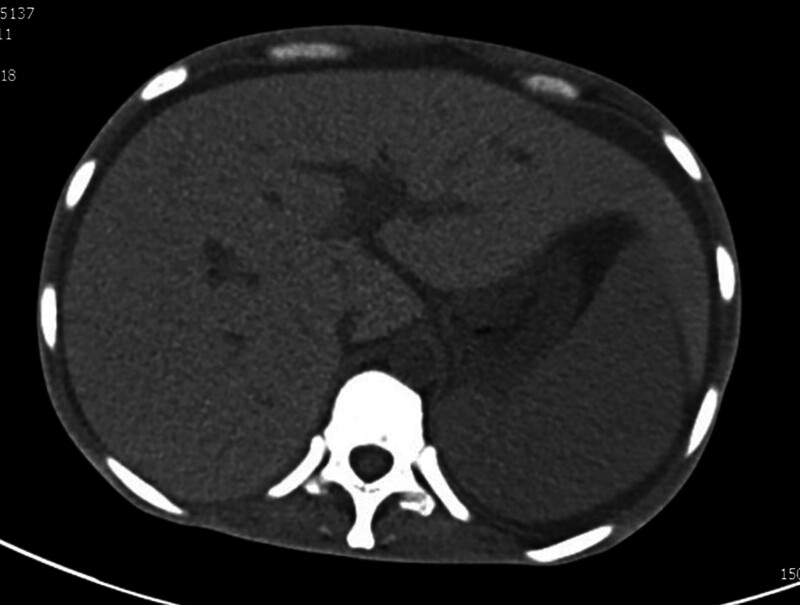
CT revealed hepatosplenomegaly. CT = computed tomography.
